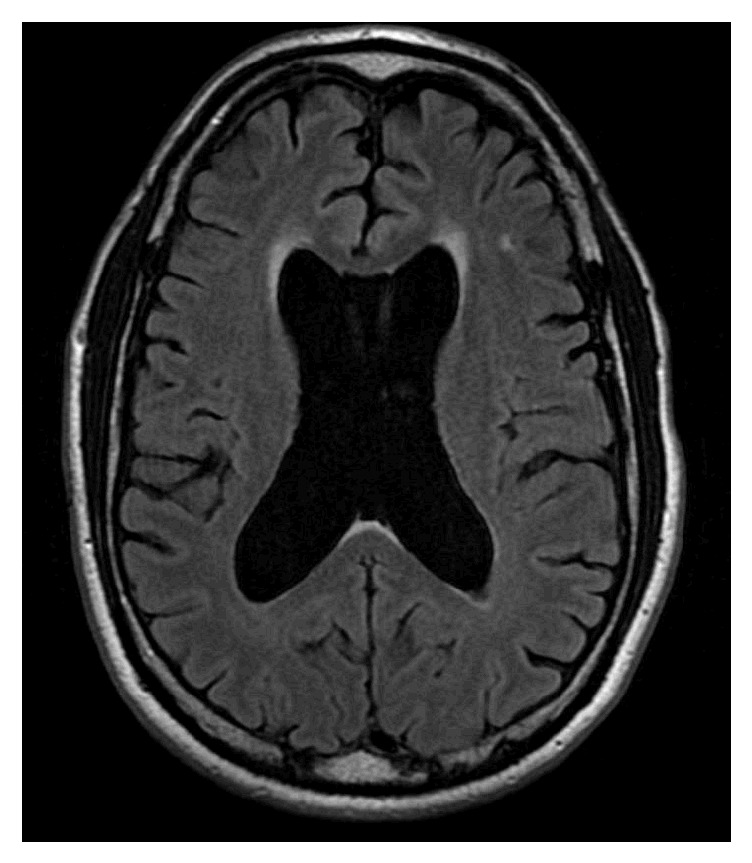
MRI reveals changes related to possible CTE. Axial image reveals both cortical and subcortical atrophy, enlarged lateral and third ventricle, and cavum septum pellucidum.
